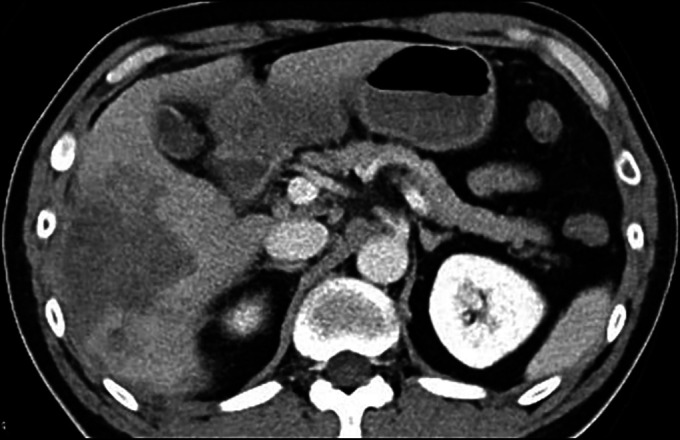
Contrast-enhanced computed tomography showing an irregular, well-defined, poorly enhanced tumor, measuring approximately 70 mm in diameter, in the right lobe of the liver.
